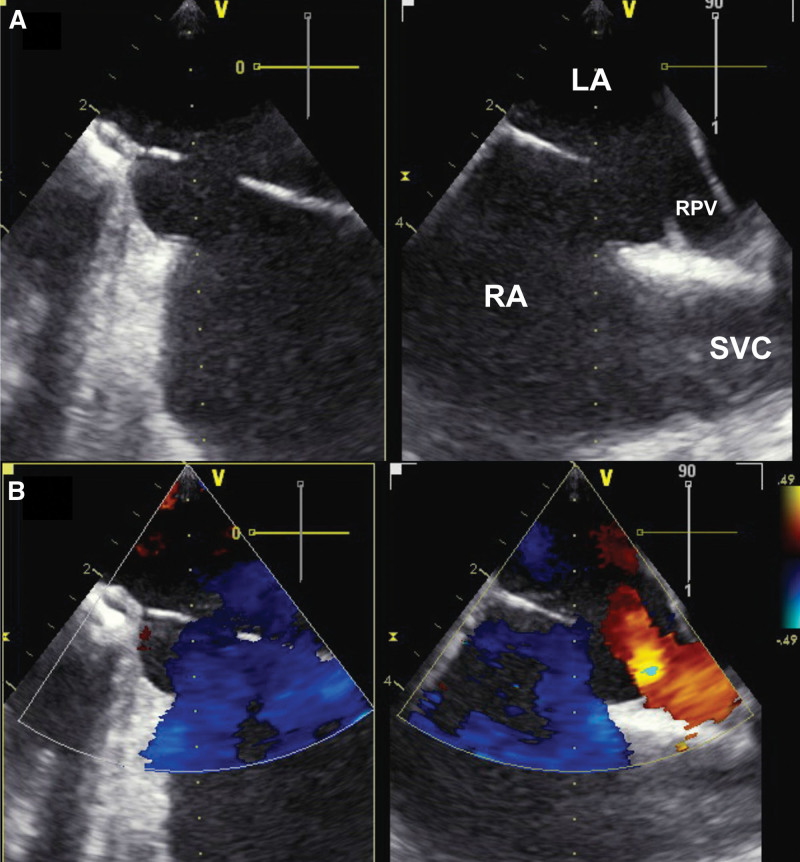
Transesophageal echocardiography of the smaller atrial septal defect. A, Biplane transesophageal echocardiography of the smaller atrial septal defect (ASD). It is located close to the arrival of the superior vena cava (SVC) and right upper pulmonary vein (RPV) precluding an interventional closure due to the risk of the obstruction of the SVC or RPV. B, Biplane transesophageal echocardiography of the smaller ASD with color Doppler. LA indicates left atrium; and RA, right atrium
